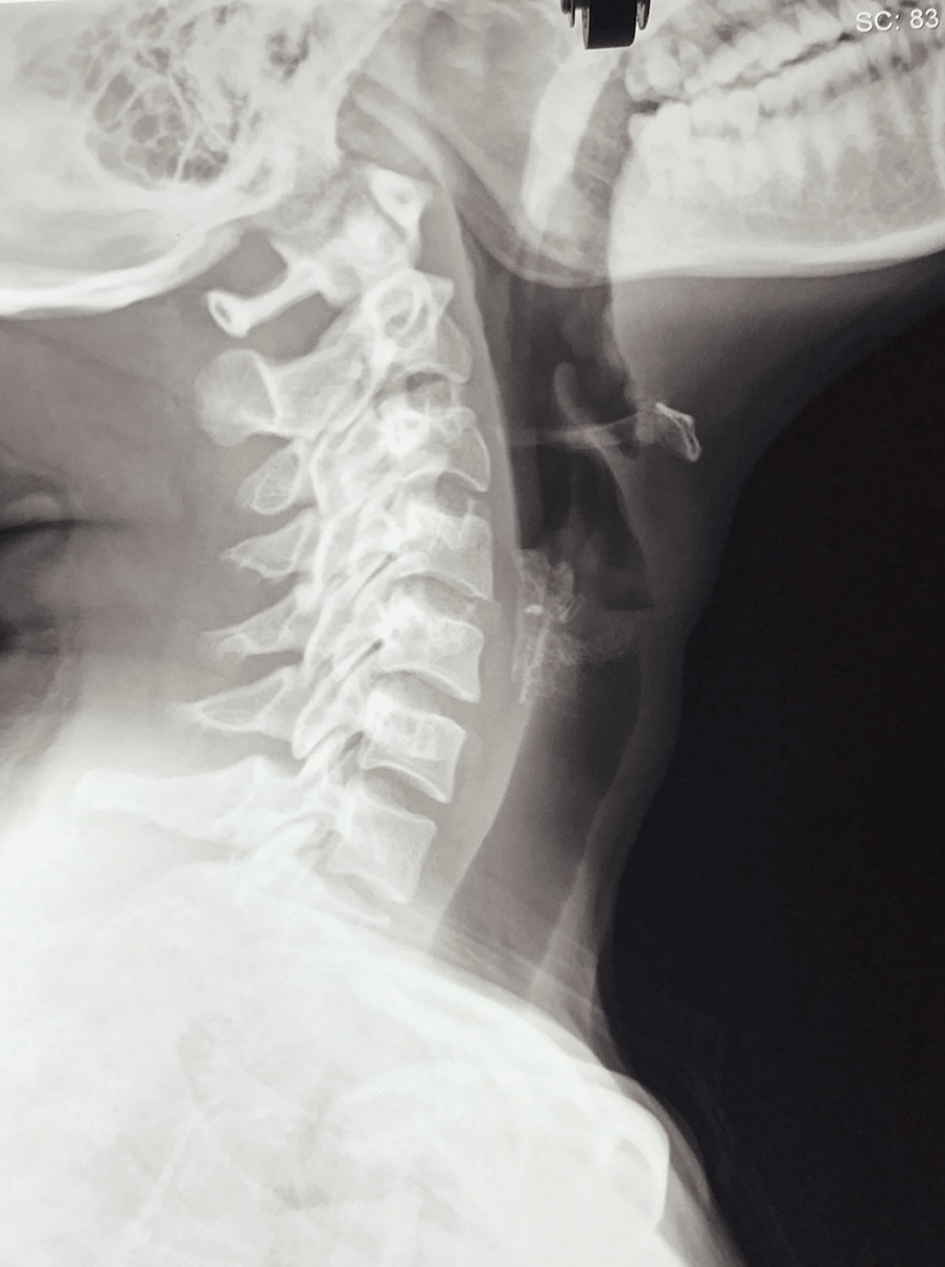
Cervical radiograph showing no abnormalities
